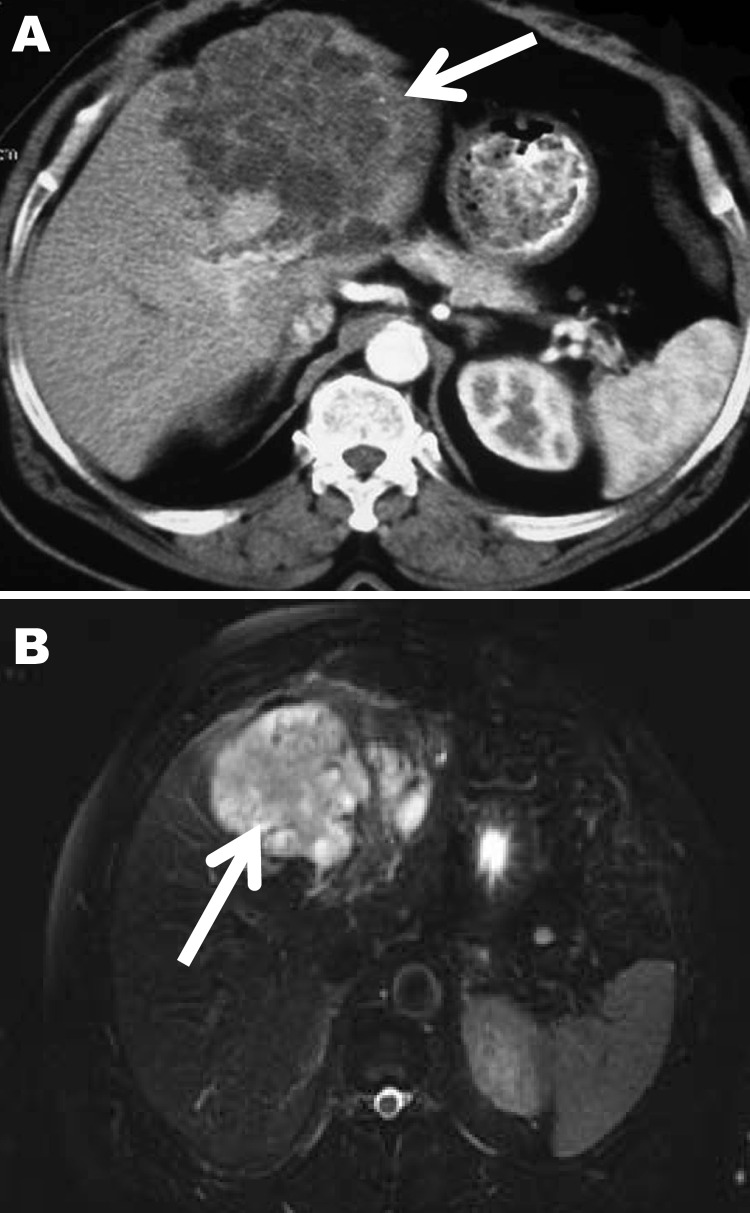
Computed tomography (A) and magnetic resonance (B) images of the liver of a 72-year-old man from French Guiana with polycystic echinococcosis affecting the left side of the liver. White arrows indicate the multicystic liver lesion.
